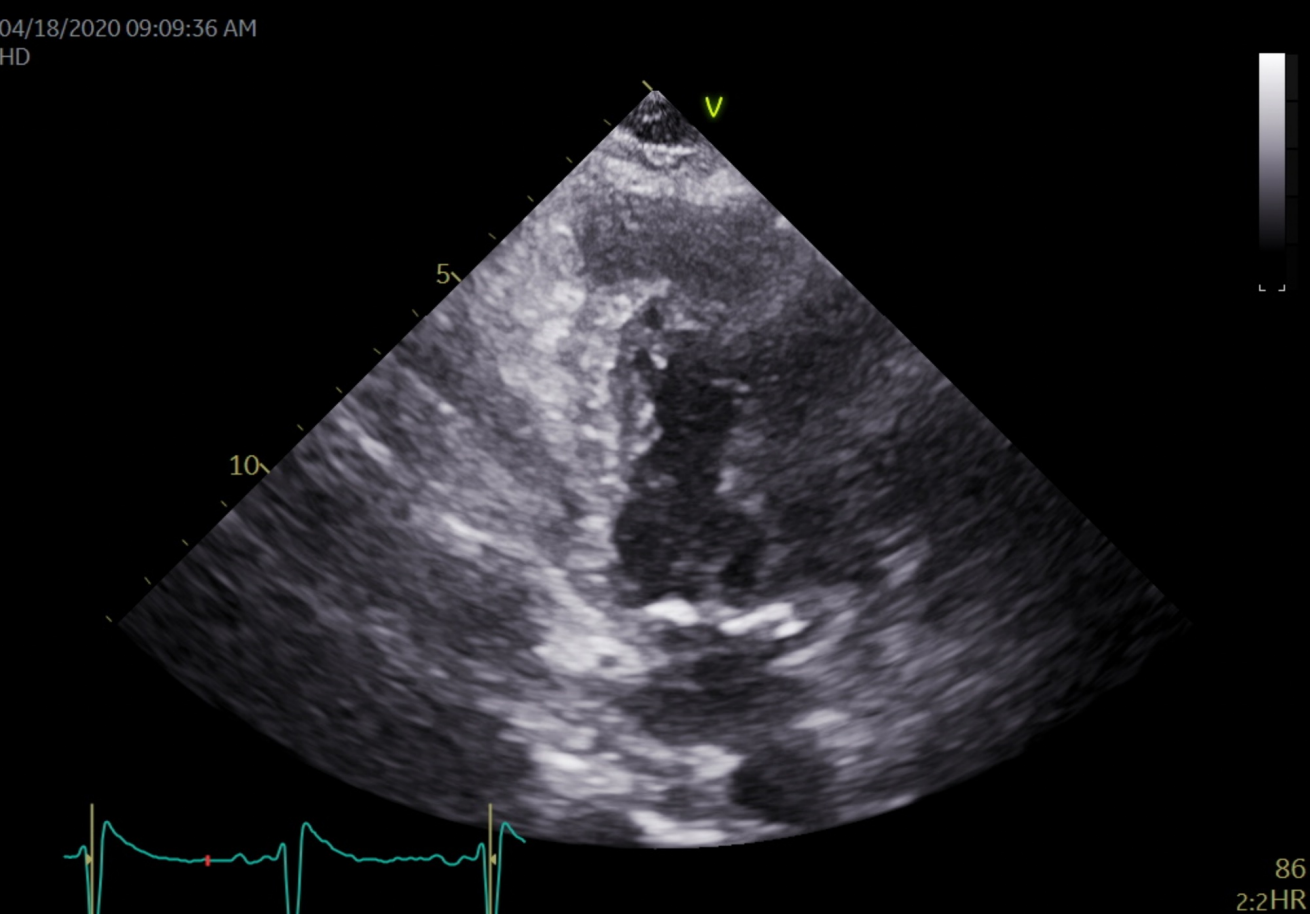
An apical 2-chamber view revealing moderate pericardial effusion is seen without evidence of tamponade
